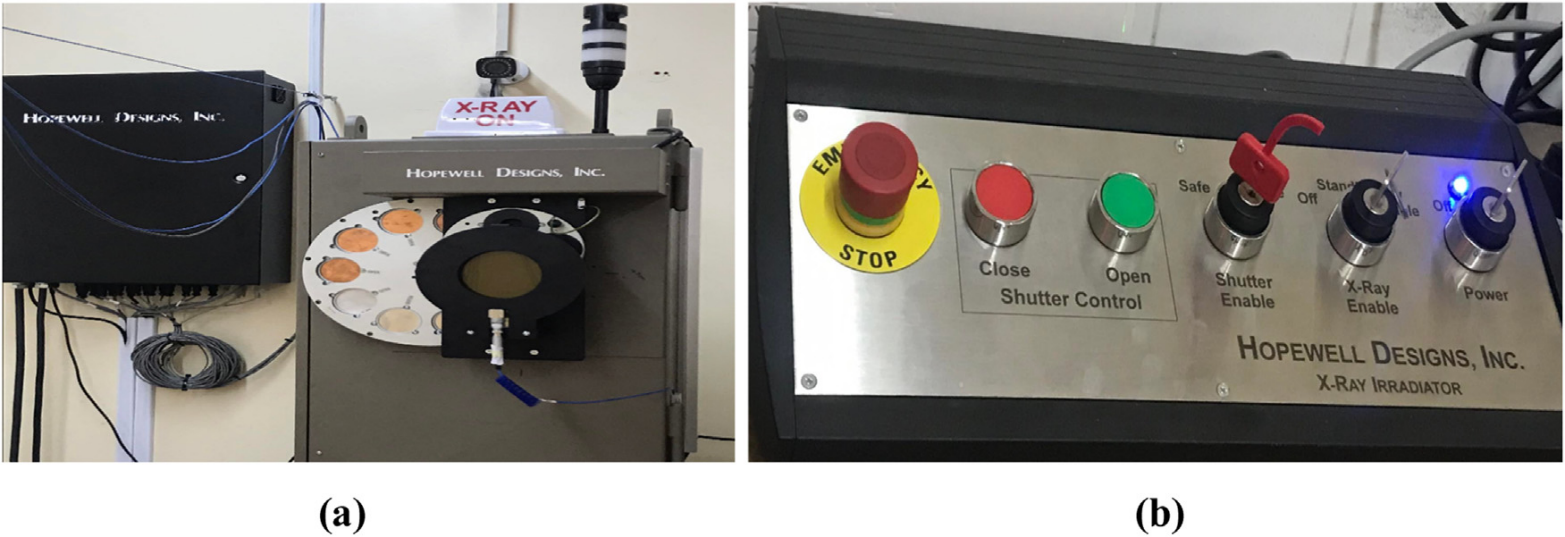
(a)* Model X80–320 kV X-ray beam irradiator used at SSDL. *(b)C*ontrol system.
